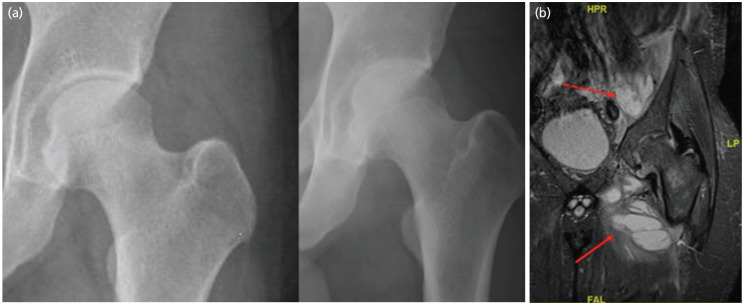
(a) Pelvis AP Radiographs two weeks apart showing acute loss of left hip joint space. (b) MRI (coronal section) showing iliacus abscess (dotted arrow) and adductor abscess (solid arrow). Evidence of destruction of femoral head cartilage and marrow enhancement.
